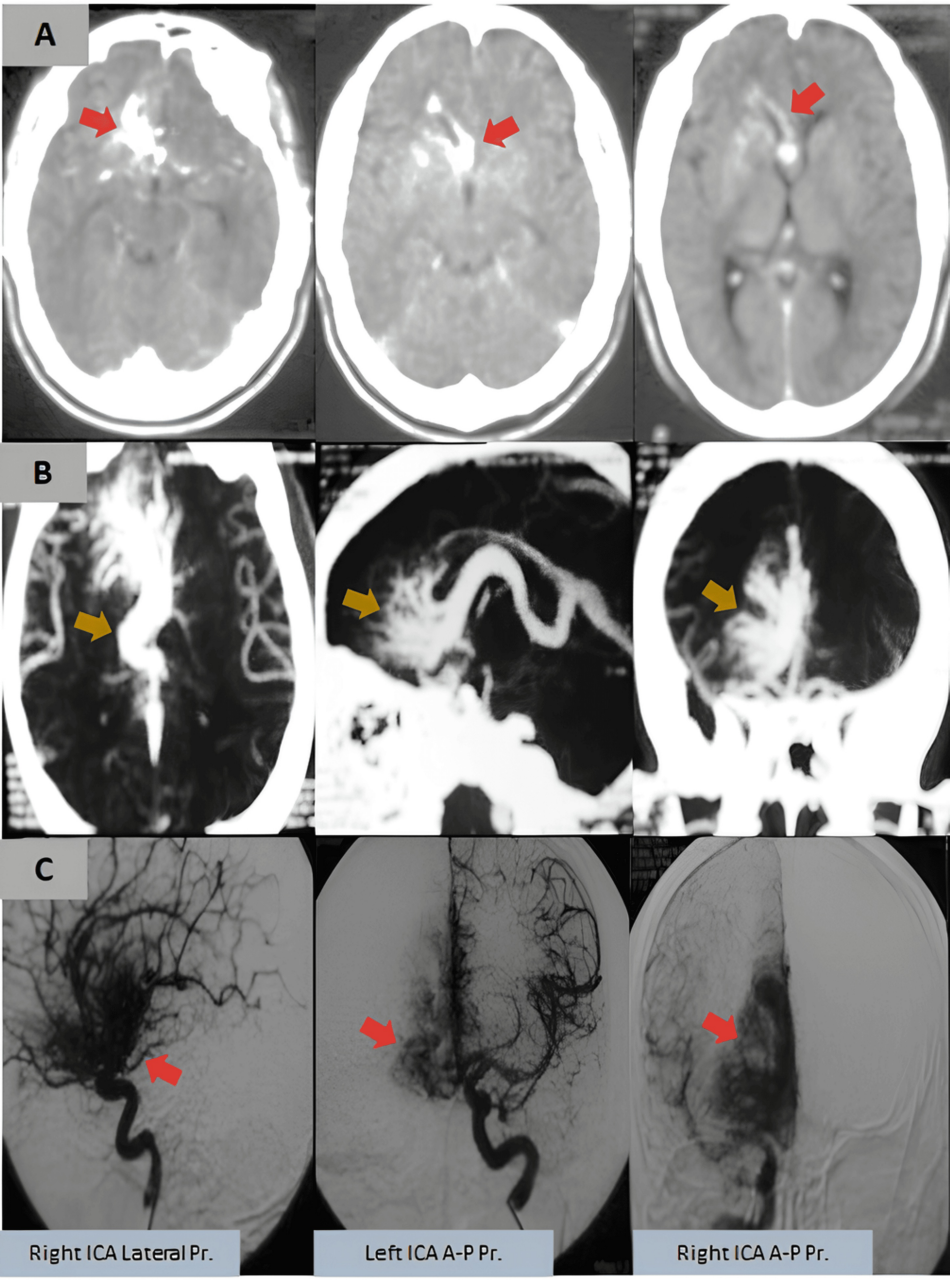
Imaging at initial presentation (A) Non-contrast CT brain images at initial presentation, revealing a subarachnoid hemorrhage in the right frontal region without intraventricular extension (arrows). (B) CT angiography and (C) digital subtraction angiography demonstrating a rare venous-predominant arteriovenous malformation mimicking an atypical developmental venous anomaly (arrows). ICA, internal carotid artery
